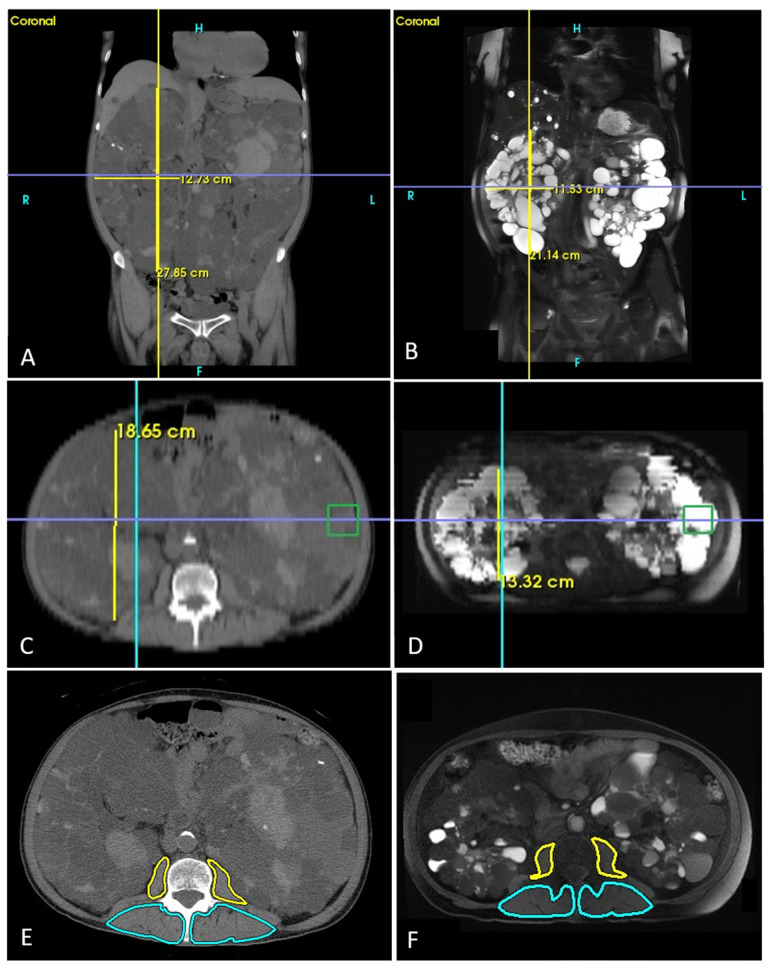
TKV was estimated, and the PM (yellow contour)/PS (blue contour) areas were measured in the same patient of pre-operative CT (A,C,E), 12-month follow-up MRI (B,D,F). TKV: total kidney volume; PM: psoas muscle; PS: paraspinal muscle; CT: computed tomography; and MRI: magnetic resonance imaging.
